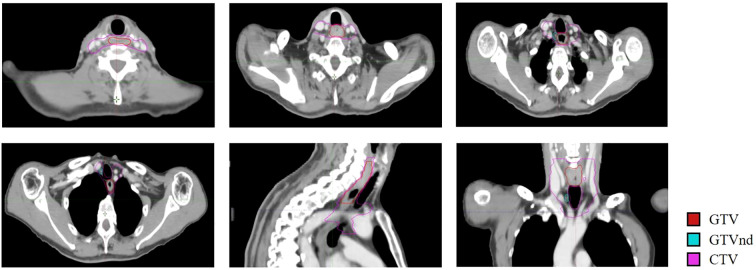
Delineation of radiotherapy target volumes. Planning CT images in multiple planes (axial, coronal, and sagittal) is shown. The gross tumor volume (GTV, red), the nodal gross tumor volume (GTVnd, cyan), and the clinical target volume (CTV, purple) are delineated. A color legend is provided.
